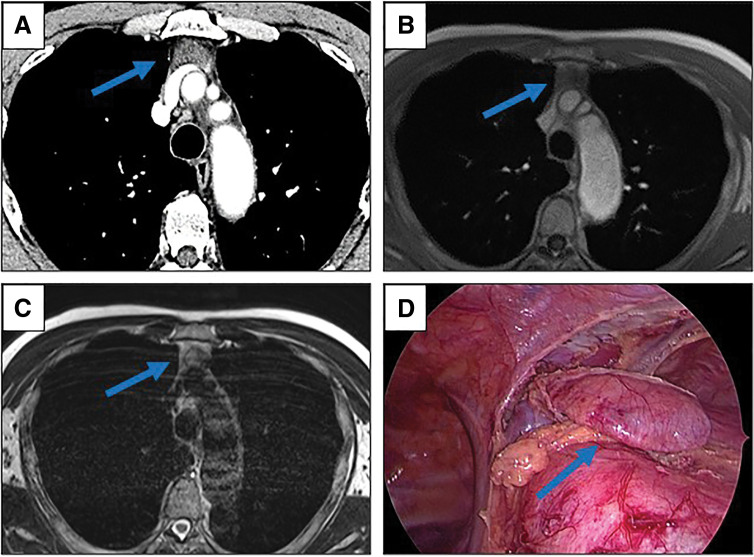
(A) Chest contrast-enhanced CT revealed an anterior mediastinal lesion with a maximum diameter of 5 cm. The lesion was slightly enhanced homogeneously (blue arrow). MRI revealed low signal intensity on T1-weighted images (blue arrow) (B) and iso-intensity on T2-weighted images (blue arrow) (C). (D) Intraoperative view. The normal thymic tissue appeared abundant for his age. The lesion did not invade the surrounding organs and was demarcated from the normal thymic tissue (blue arrow).
